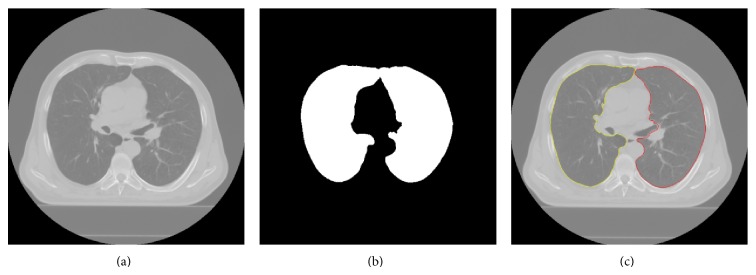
The right and left lung separation: (a) original CT slice, (b) connected lungs in white, and (c) right lung contour in yellow and left lung contour in red superimposed on the original slice.
